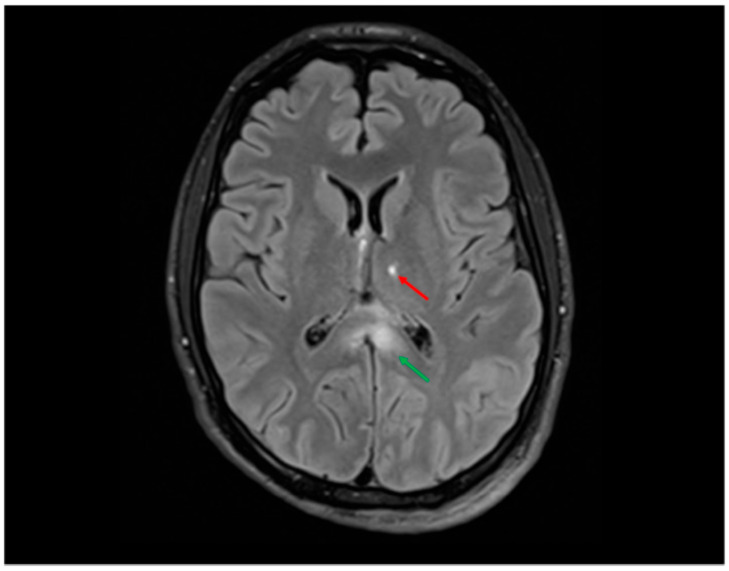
FLAIR sequence showing hyperintensities in the splenium of the corpus callosum (≈12 mm, green arrow) and at the left thalamo-capsular junction (≈9 mm, red arrow) in a young man involved in a high-speed motor vehicle collision (MVC). These locations are typical of grade II DAI according to the Adams classification and are frequently associated with impaired consciousness, cognitive deficits, and motor dysfunction. In this case, the patient presented with prolonged coma in the acute phase, in line with the neuroradiological findings.
